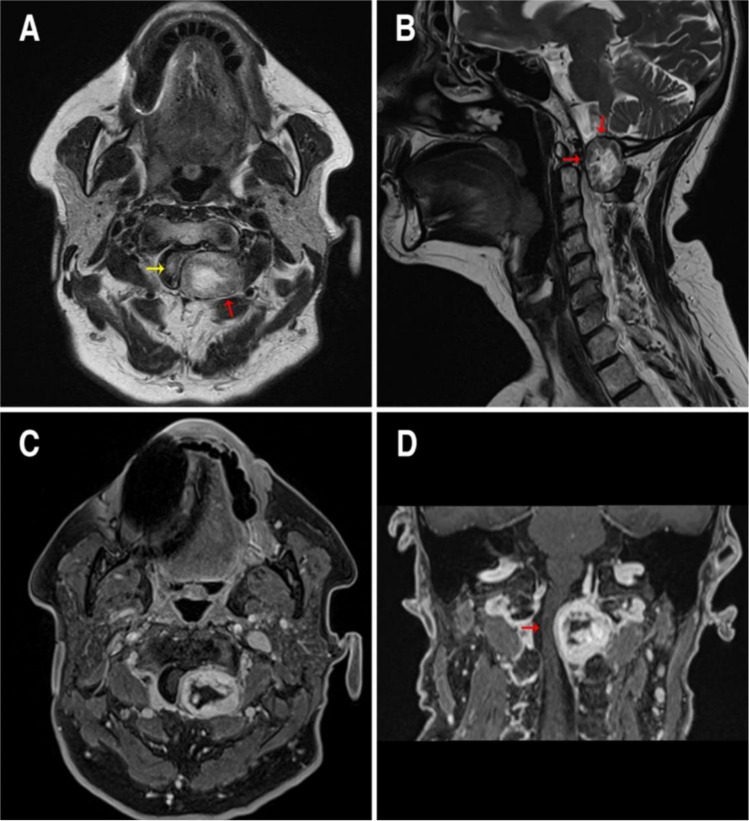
MRI images showing a left cervical extra-axial expansive lesion (22 × 28 × 32 mm) with intra- and extracanalicular extension at the C1–C2 level and occipital extension through the foramen magnum (red arrows). A Axial T2-weighted MRI view highlighting the intracanalicular extension of the lesion and altered signal in the cervical spinal cord, indicating compressive myelopathy (yellow arrow). B Sagittal T2-weighted MRI view showing the tumor’s intracranial extension. C Axial T1-weighted MRI view demonstrating the encapsulated nature of the tumor. D Coronal MRI reconstruction showing spinal cord compression and rightward displacement
